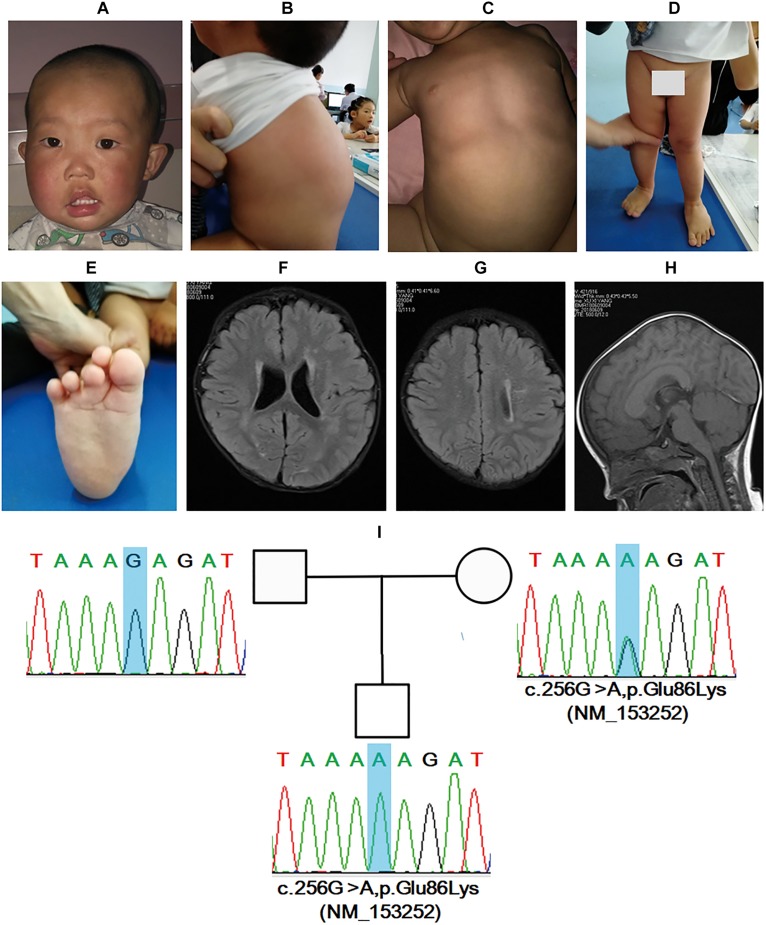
BRWD3* variant induces mental retardation, X-linked 93 in a 1-year-and-8-month-old male patient. Facial dysmorphic features, including asymmetry, high forehead, hypertelorism, downturned corners of mouth, strabismus, drooling, and protruding ears were noted (A). Multiple bulging masses in the back, slight pectus excavatum, lower limb asymmetry, and pes planus were revealed (B–E). Head MRI showed abnormalities in bilateral frontal and parietal white matter together with corpus callosum (F–H). Sanger sequencing demonstrated that the boy had a novel mutation inherited form his mother (I).
